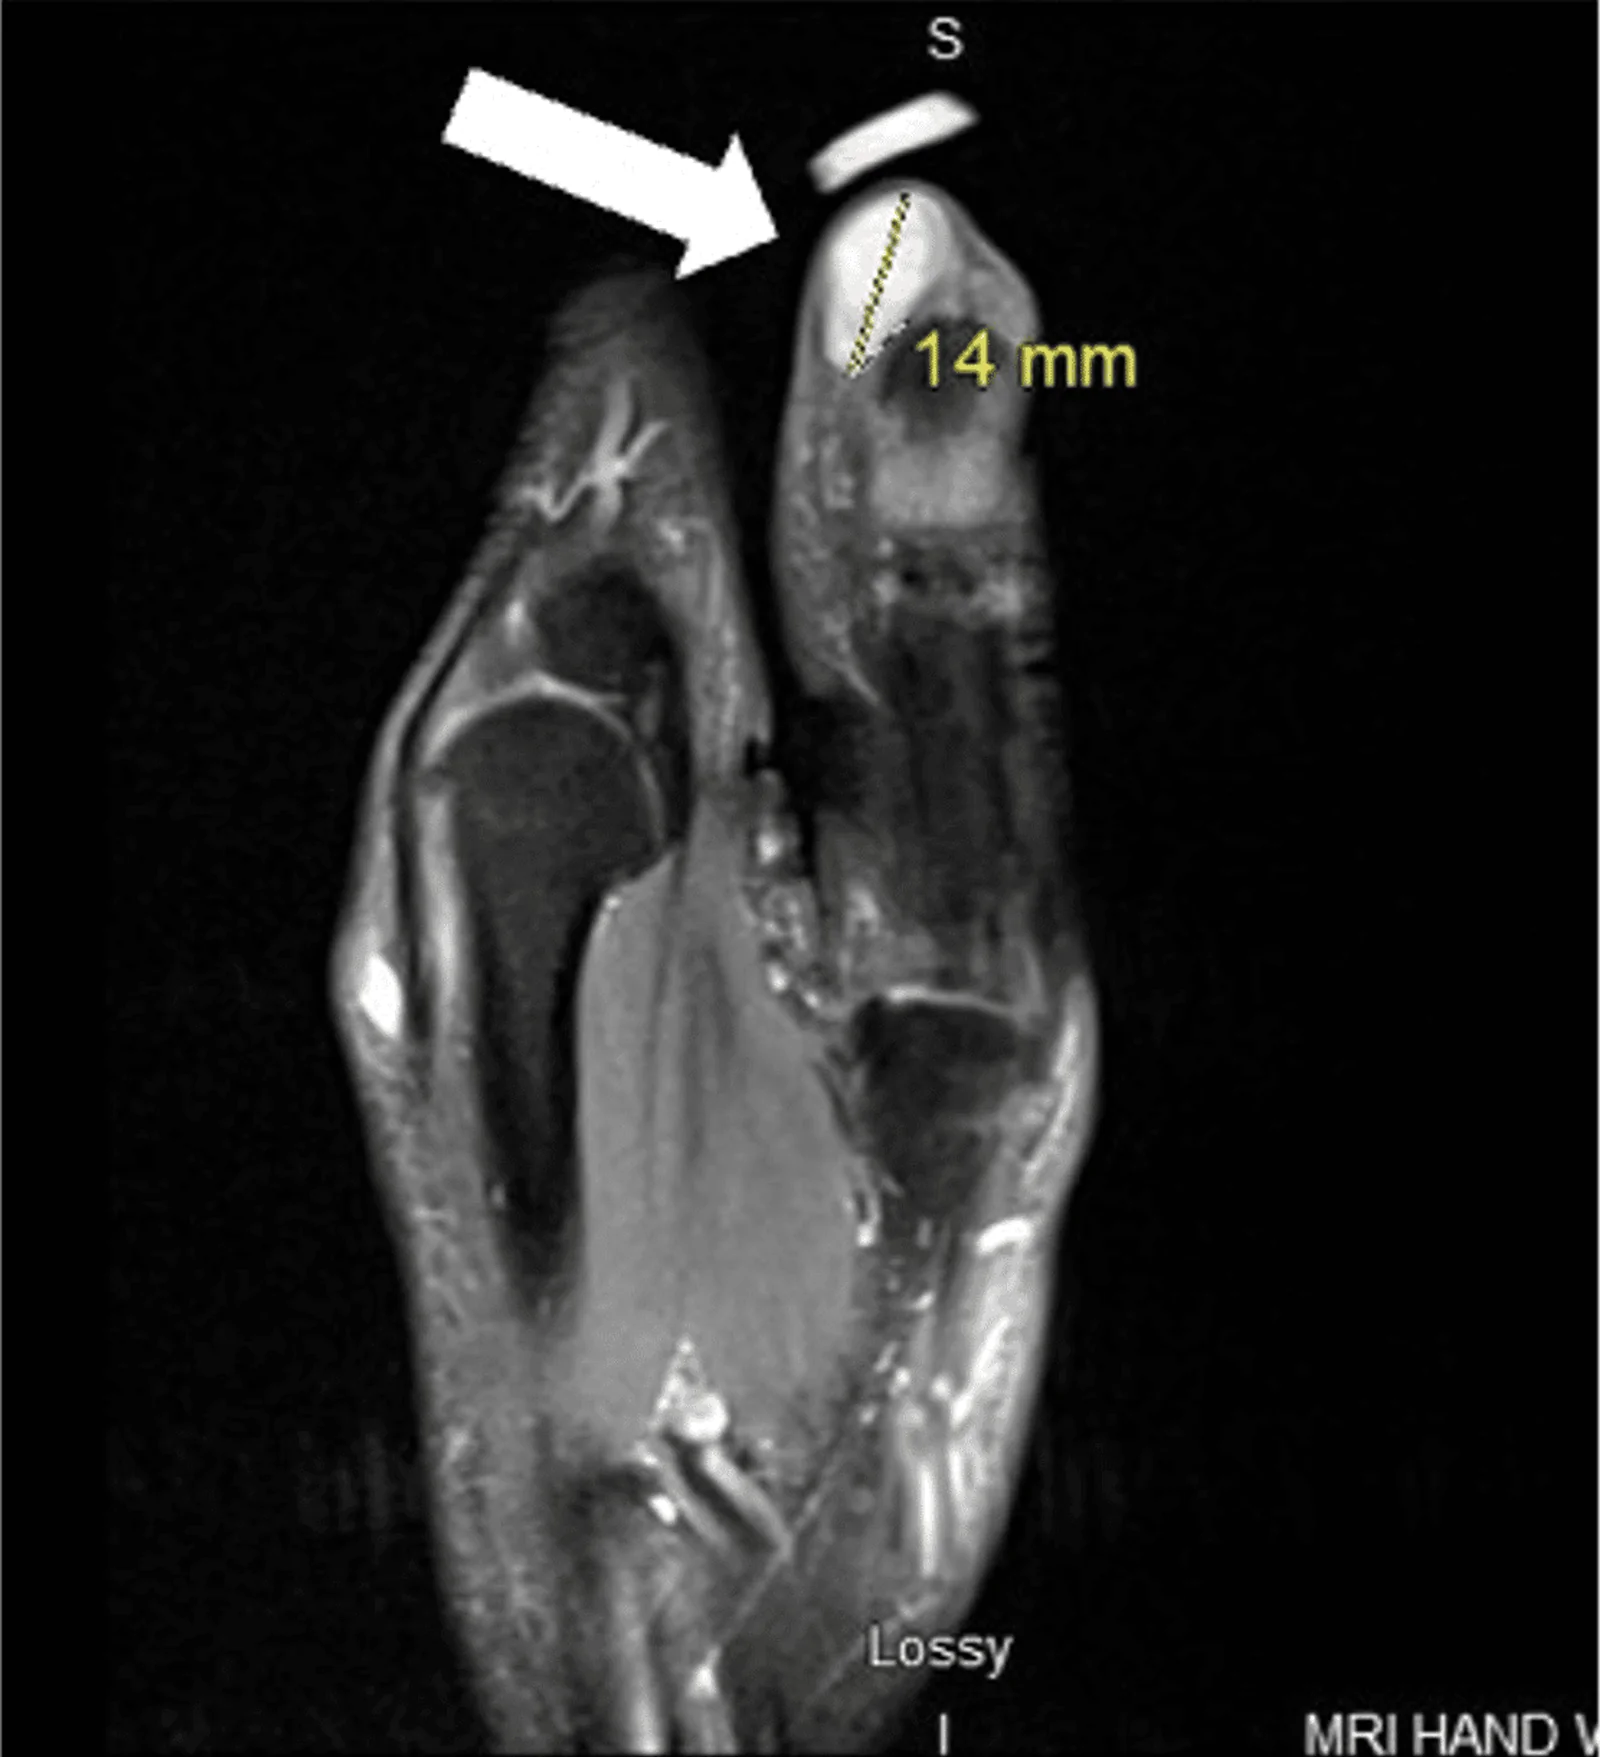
Axial PD FS magnetic resonance image of the right thumb demonstrating a well-circumscribed, homogeneous, high-signal-intensity lesion within the thumb pulp, contributing to its cystic appearance. PD FS: proton-density fat-saturated
